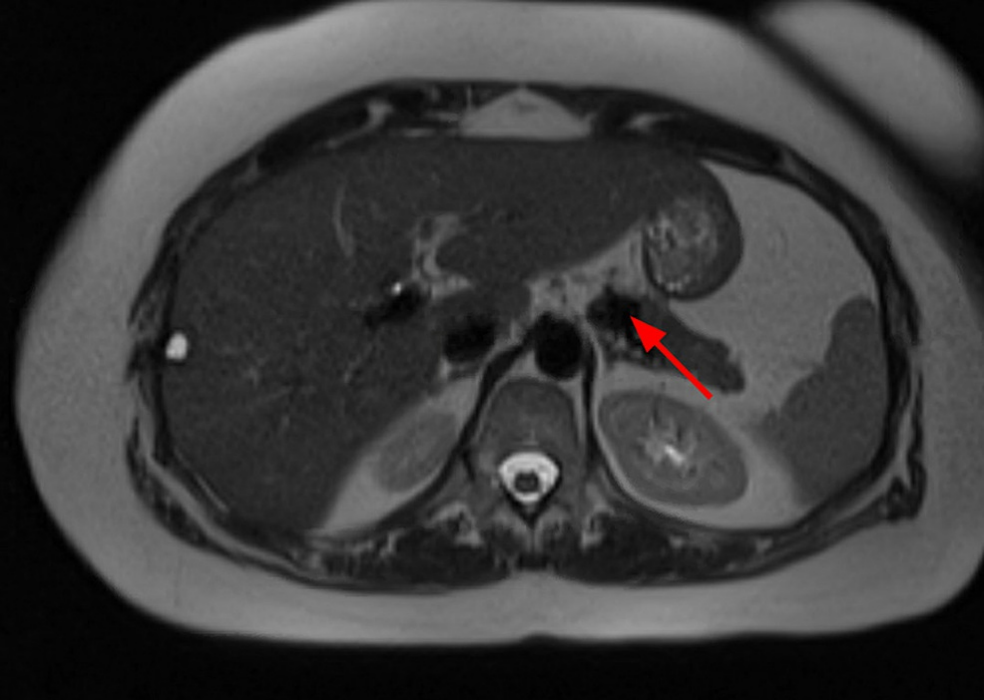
MRI showing a T2-hypointense lesion adjacent to the pancreatic body (red arrow), favored to represent an SAA. SAA, splenic artery aneurysm
